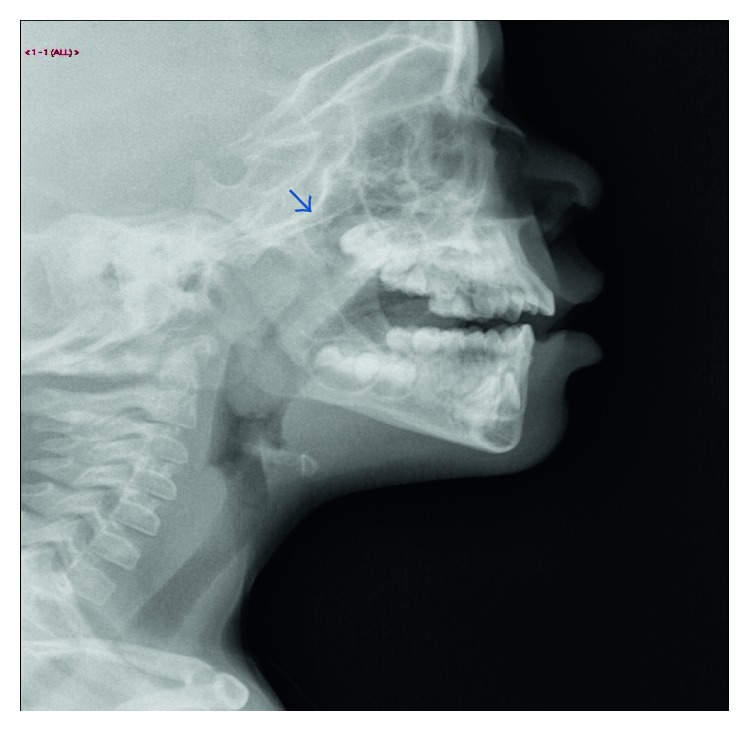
Soft tissue X-ray image showing adenoid enlargement.
